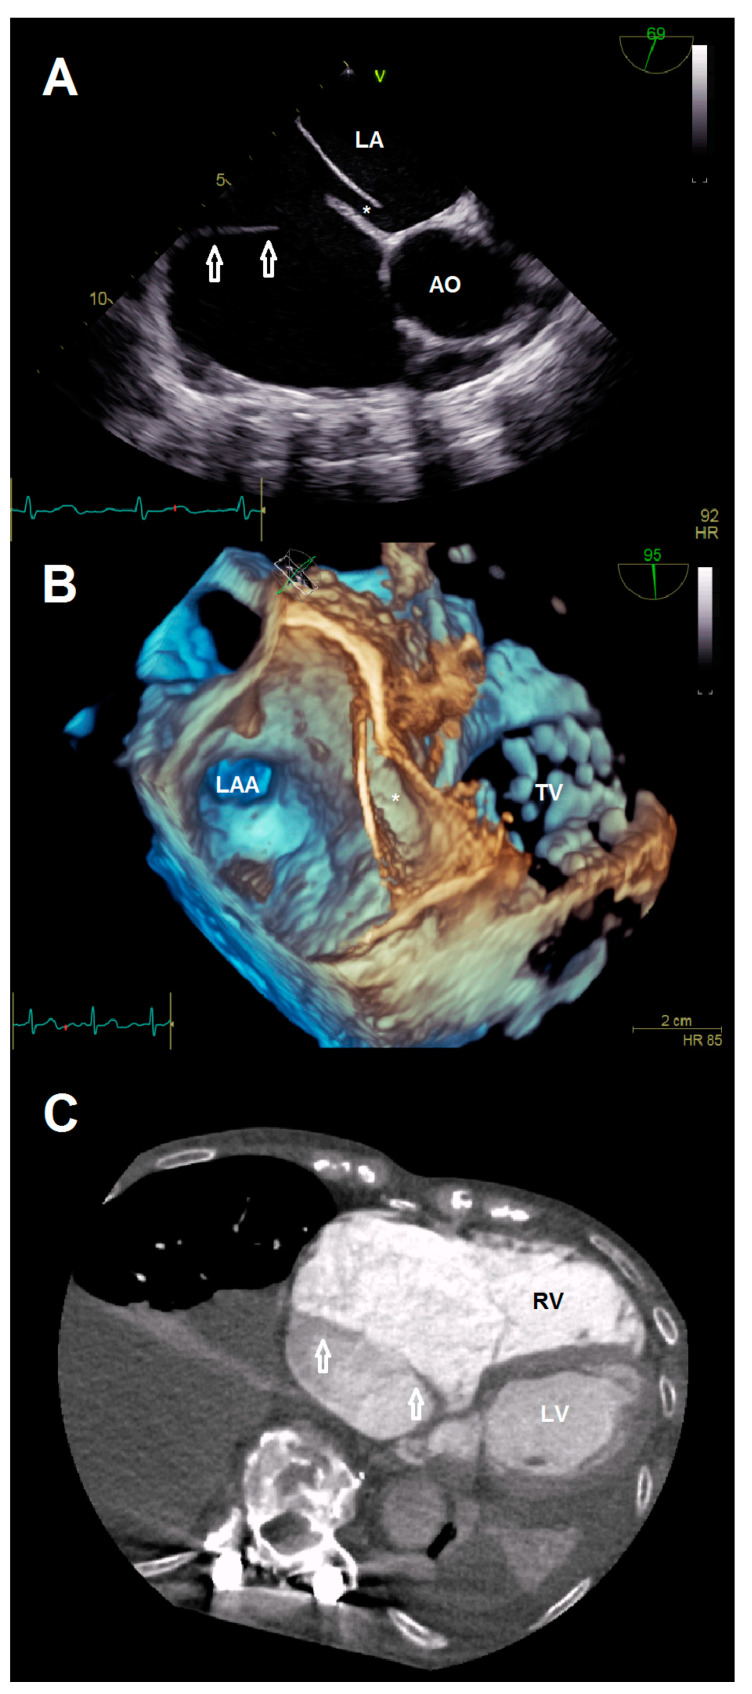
(A) Anatomical relationship between the additional membrane (arrows) and present patent foramen ovale (*). (B) 3-D view showing the patent foramen ovale (*). (C) Cardiac computed tomography visualizing the cor triatriatum dexter (arrows) for procedural planning. Abbreviations: AO, aorta; LA, left atrium; LAA, left atrial appendage; LV, left ventricle; RV, right ventricle; TV, tricuspid valve.
